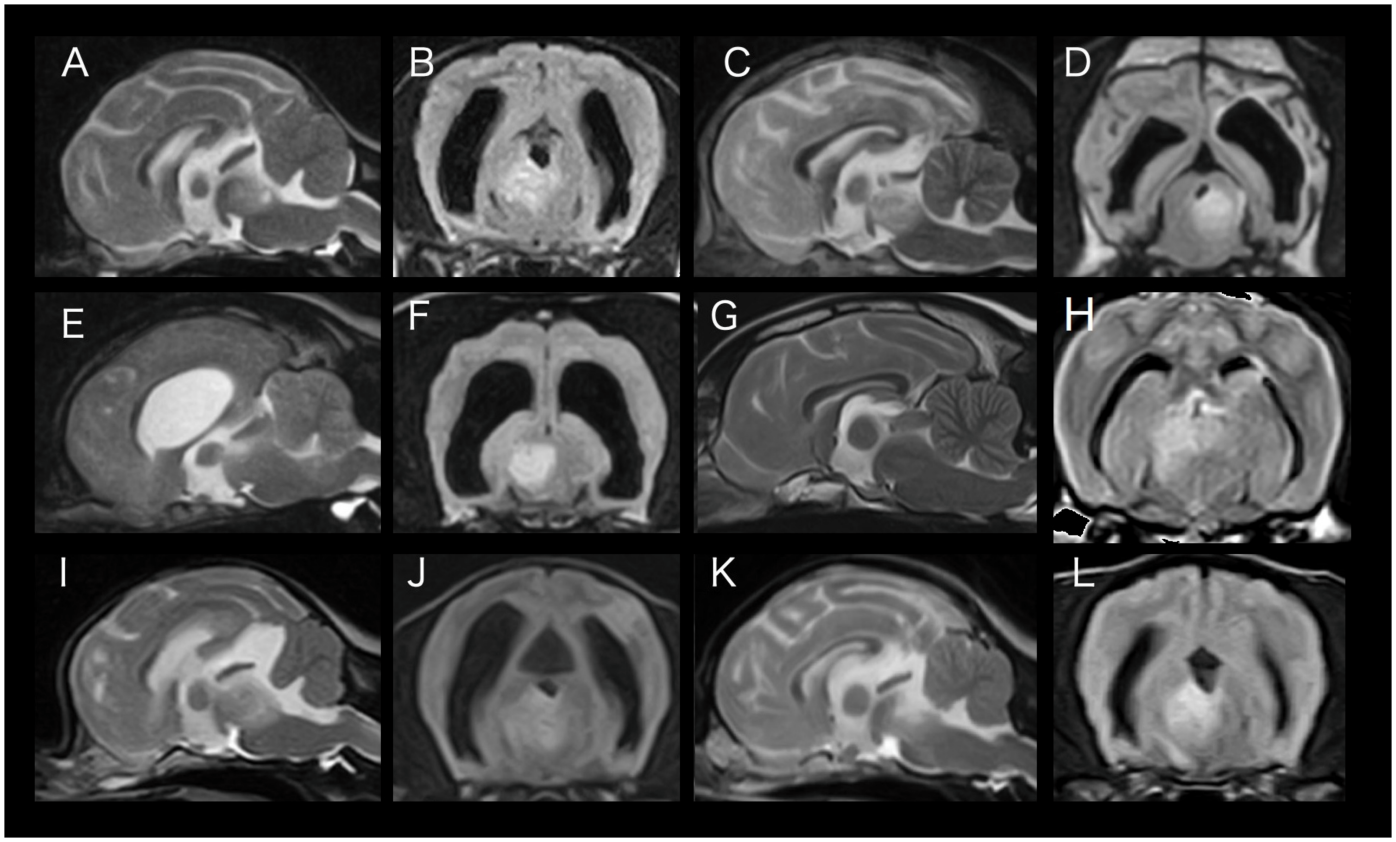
T2-weighted mid-sagittal magnetic resonance images (A: case 1, C: case 2, E: case 3, G: case 4, I: case 5, K: case 6) and fluid attenuated inversion recovery transverse images at the level of the midbrain (B: case 1, D: case 2, F: case 3, H: case 4, J: case 5, L: case 6). There were additional lesions elsewhere (multifocal) other than what is indicated here (see descriptions in the text per case). Lesions in the midbrain were deemed asymmetrical in all cases.
